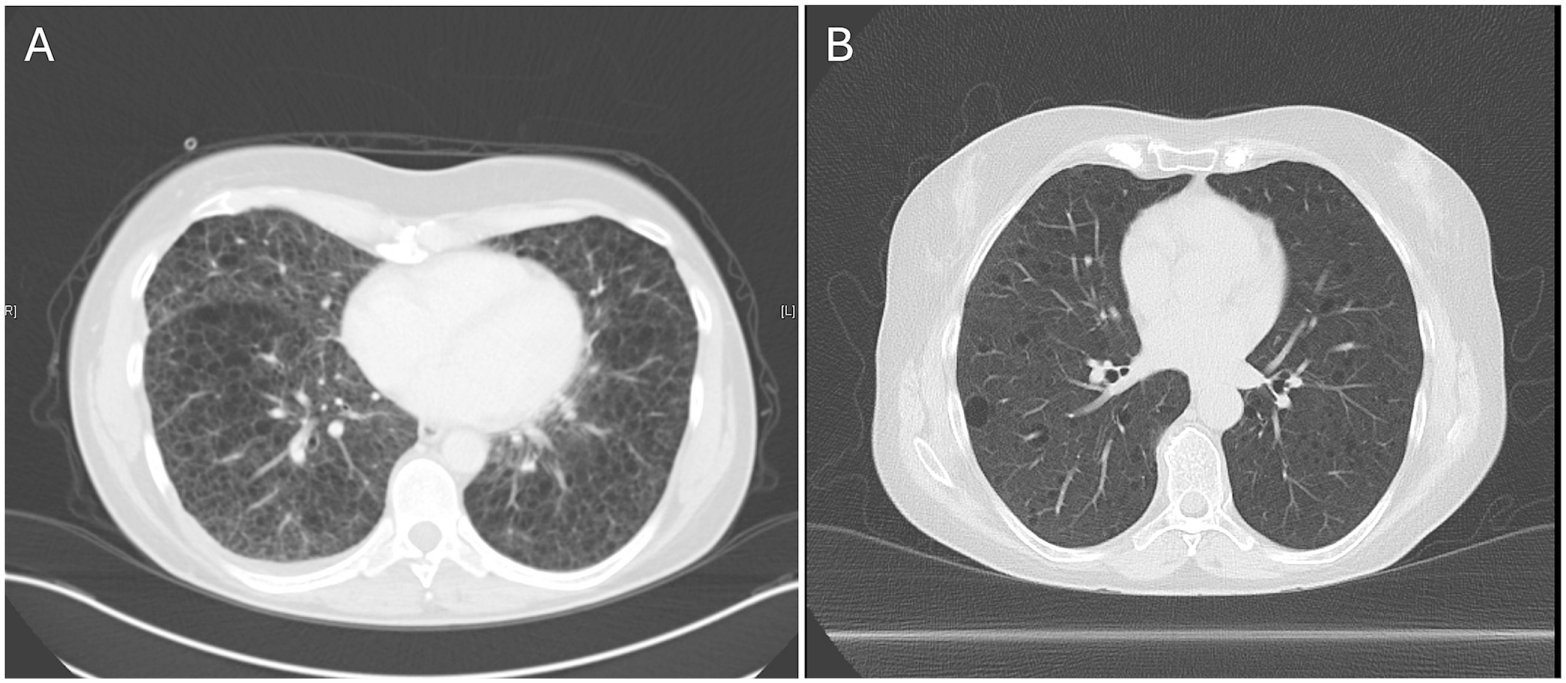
Representative lung CT-scans of STLAM1 and STLAM7. (A) CT images from STLAM1, with a high allelic frequency (99%) of the FGFR4 p.Gly388Arg polymorphism, demonstrate advanced cystic destruction. (B) In contrast, STLAM7, with no FGFR4 p.Gly388Arg polymorphism, exhibits bilateral cysts of varying sizes alongside preserved lung areas.
